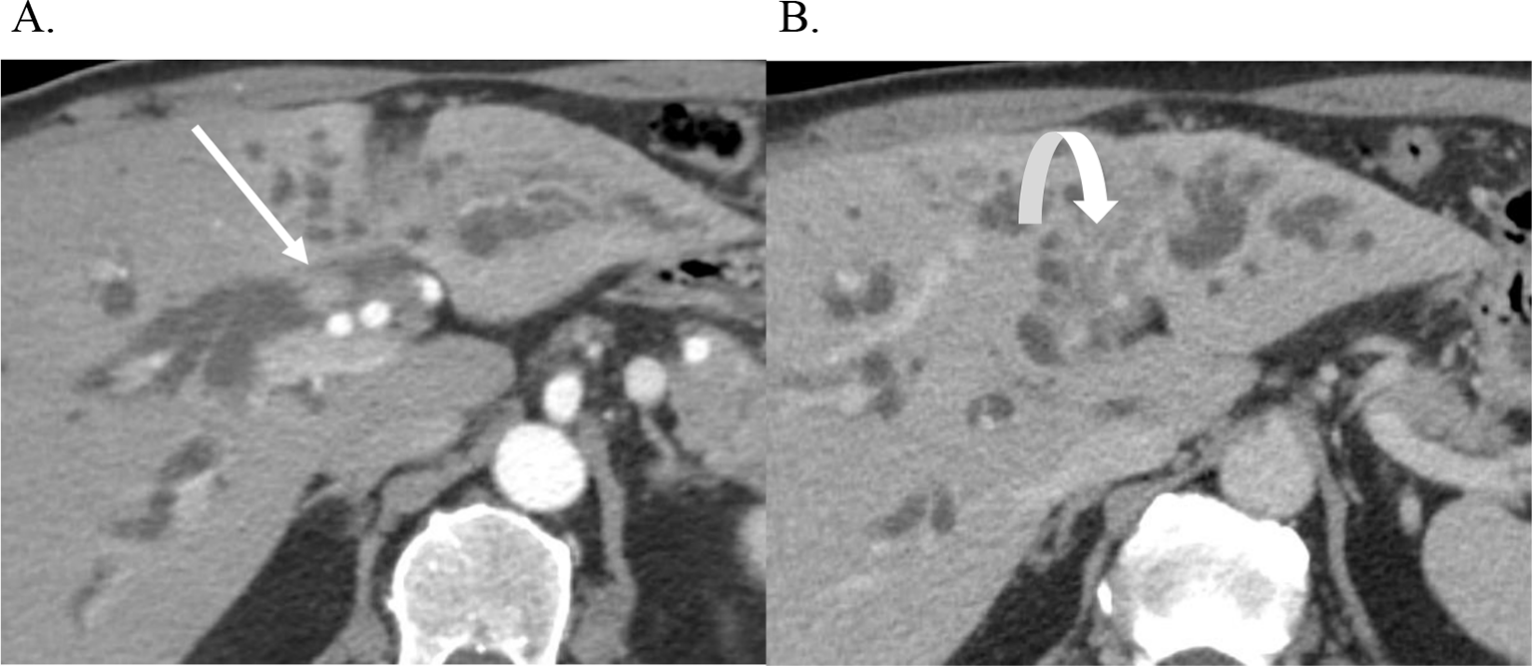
Intraductal CCA. Axial CT images with contrast demonstrate an intraductal perihilar mass (arrow) with upstream intrahepatic ductal dilatation (A). There is tumor thrombus in the left portal vein (curved arrow) (B)
